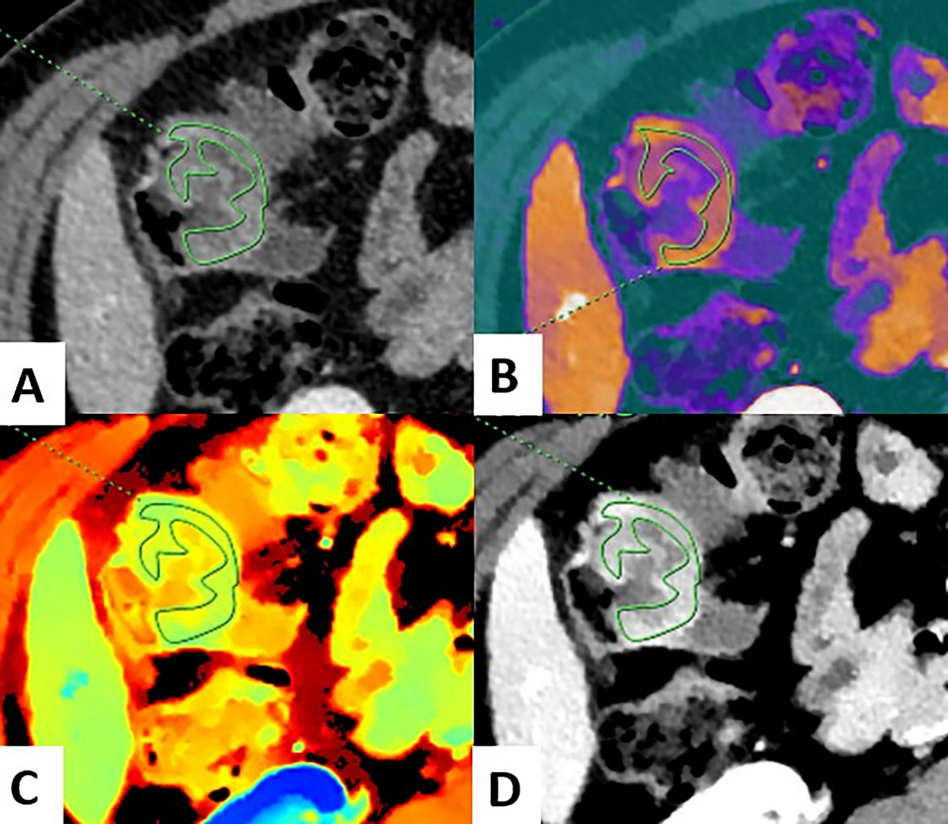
58-year-old male patient with a right pT3N2 right-sided colon cancer. DLCT (Iqon, Philips) in axial section at VP with manually outline ROI. A Conventional CT. B Iodine map. C Z-eff map. D 40 keV VMI. CRC, colorectal cancer; ROI, region of interest; VMI, virtual monoenergetic images; VP, venous phase; Zeff, Z-effective
